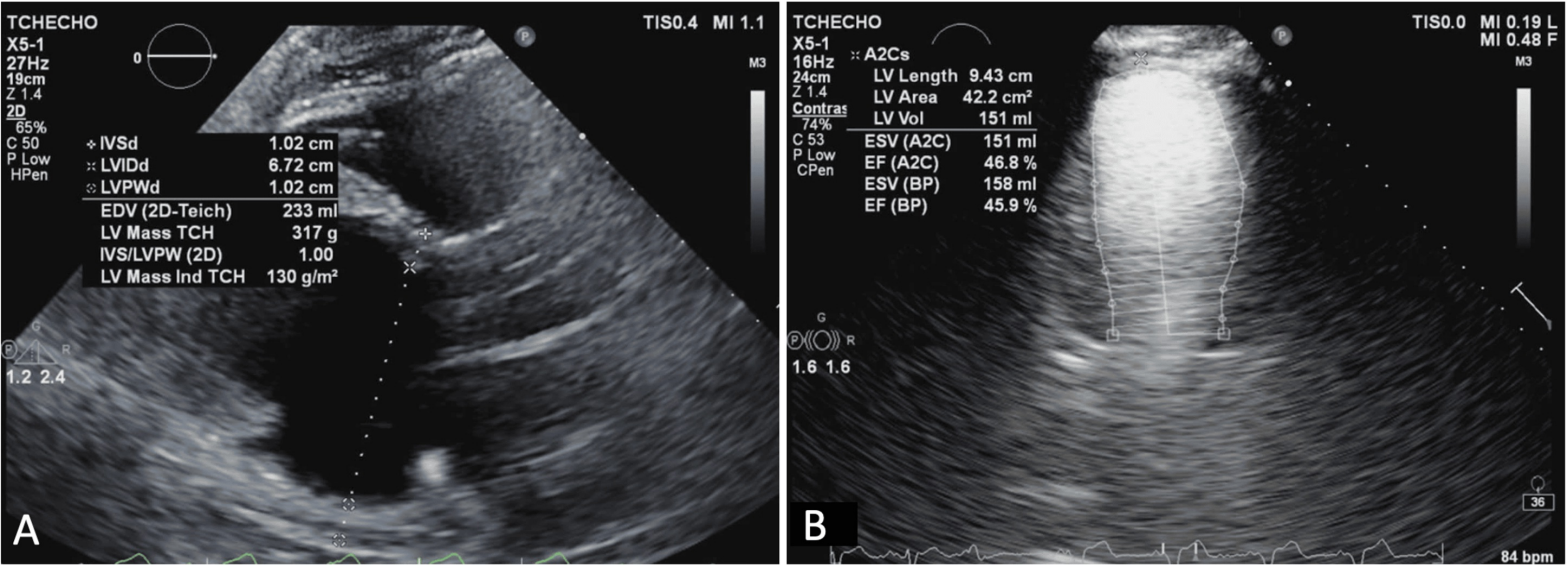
Parasternal long-axis (A) and two-chamber view (B) TTE demonstrate eccentric hypertrophy (normal LV wall thickness and increased mass index). An increased end-diastolic diameter measuring 6.7 cm is noted. Improvement in LVEF at the time of repeat echo is now calculated at 46% by Simpson’s biplane method. TTE: transthoracic echocardiography; LV: left ventricular; LVEF: left ventricular ejection fraction
